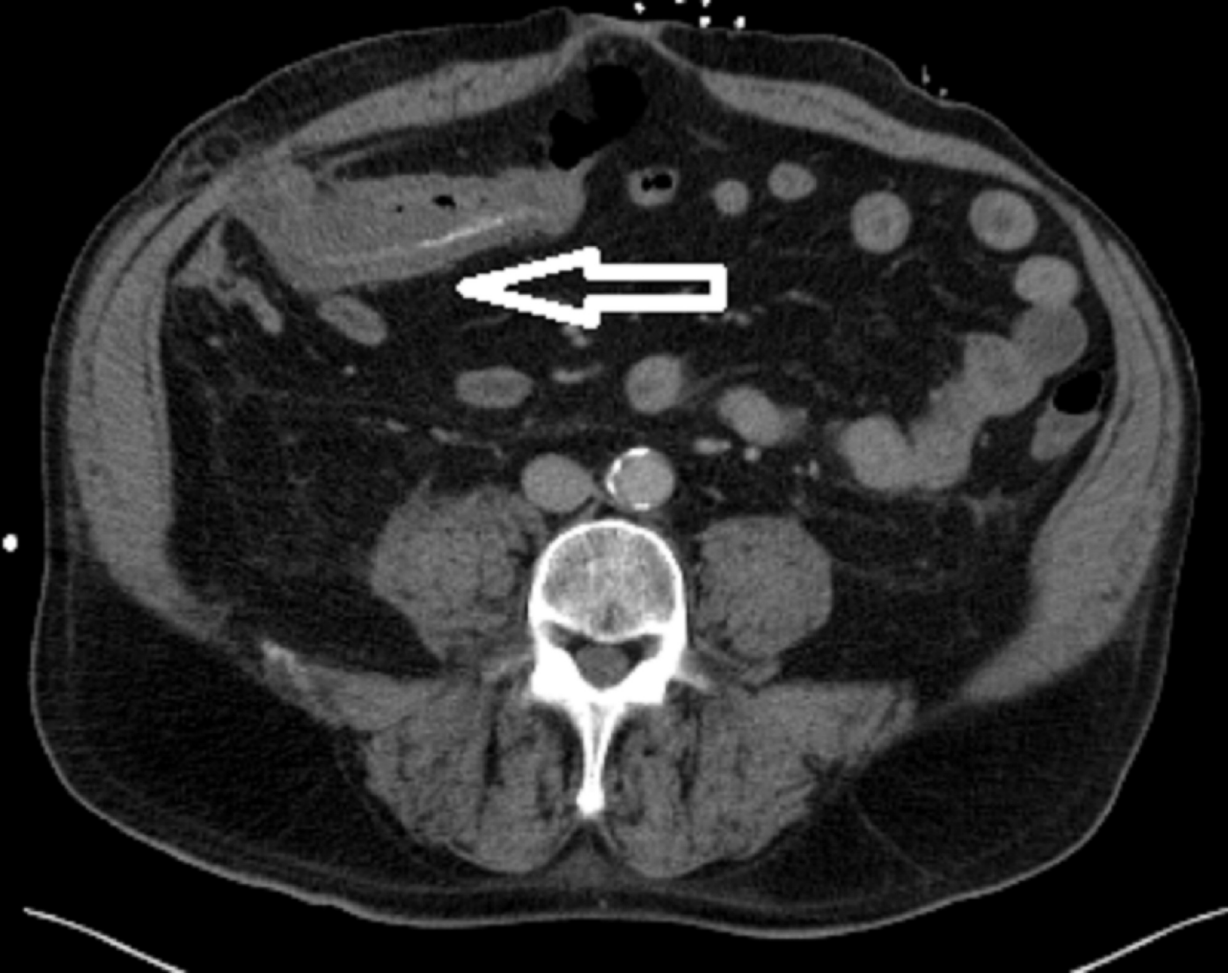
Follow-up computed tomography (CT) scan after clinical decompensation. CT scan showing persistent colonic wall thickening over a 10 cm segment of transverse colon. This thickened segment of colon was previously contained in the Spigelian hernia from Figure 1. The white arrow shows the hernia which is now reduced in relation to Figure 1.
